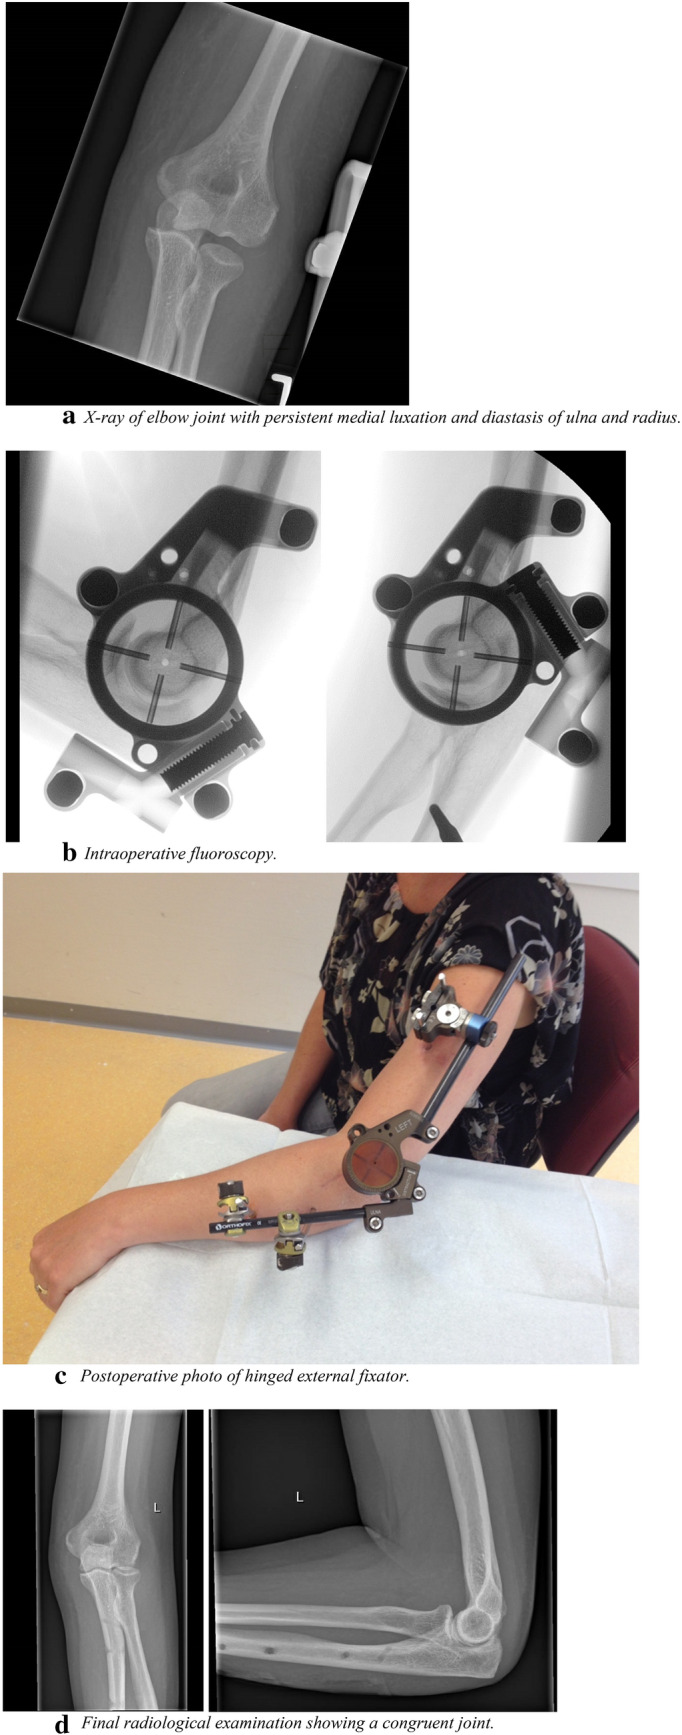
Radiological findings of a typical case. Hereby we present a case from a patient with a simple elbow dislocation with persistent luxation after external fixation elsewhere, after a low-energy trauma caused by a fall on March 8th, 2012. a X-ray of elbow joint with persistent luxation and diastasis of ulna and radius. We removed the static fixator, performed an extended arthrolysis and anatomic reduction of the elbow joint, followed by applying a dynamic hinged elbow fixator on May 15th, 2012 (68 days after trauma), resulting in congruent elbow joint in flexion and extension during surgery. b Intraoperative fluoroscopy images. c Postoperative photo of hinged external fixator. After 8 weeks, the dynamic fixator was removed. d The treatment resulted in a congruent anatomically joint seen on final radiological examination. At last follow-up clinical result was excellent with a flexion–extension arc of 140° and pronation-supination arc of 180°. The postoperative course was uneventful, and the patient was discharged from follow-up after 7.3 months
